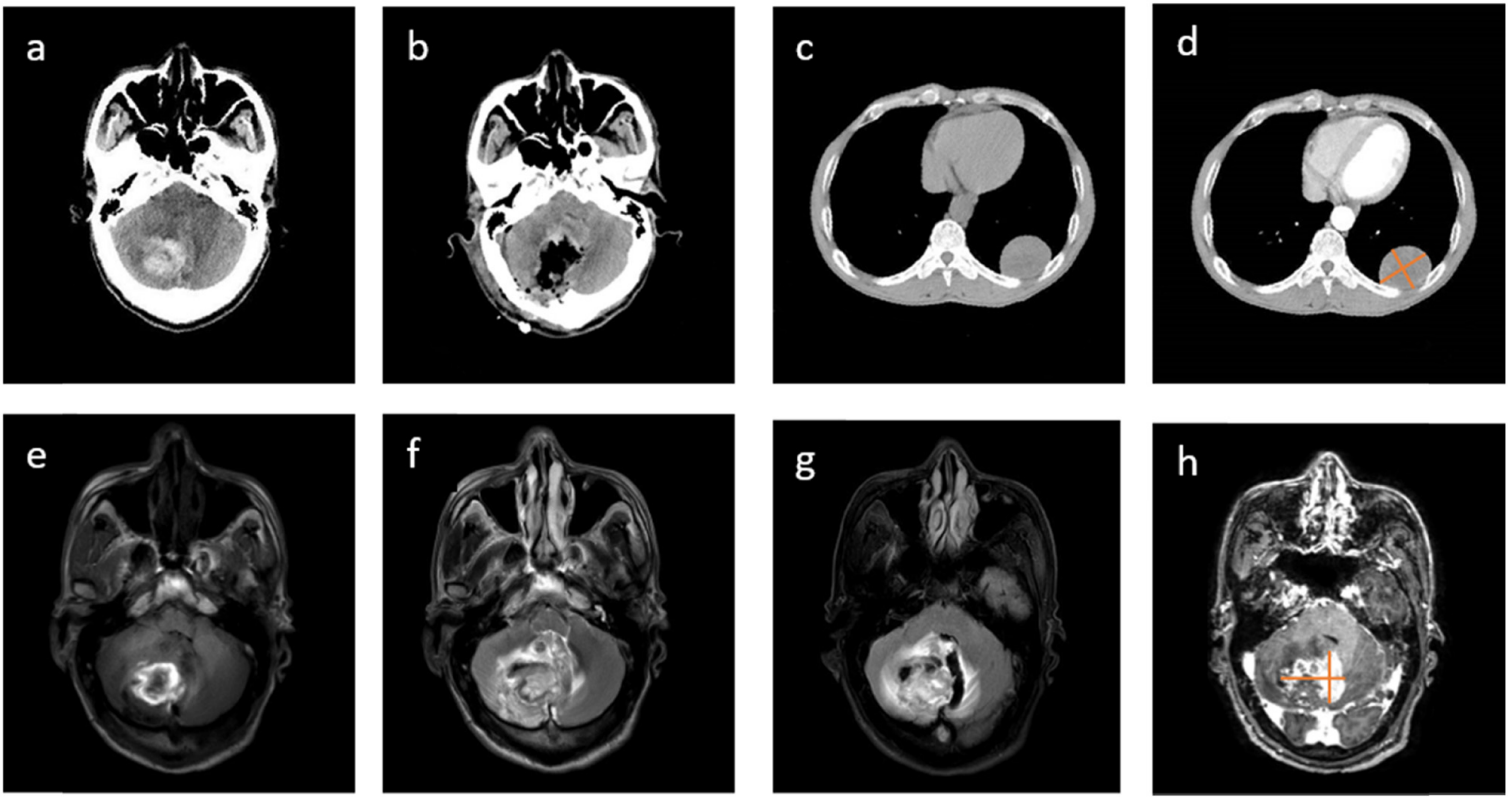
CT scan of the emergency room showed hemorrhage in the right cerebellar hemisphere (a). Postoperative CT scans revealed clear axial plane views of the surgically cleansed meningiomas and hematomas (b). (c) An image of a subpleural mass in the left lower lobe with well-defined, well-intact margins, and a slightly less uniform density. After enhancement scanning, there is a little heterogeneous enhancement of the chest occupation, tumor size is about 3.4 cm × 3.2 cm (d). The right cerebellar hemisphere exhibits a patchy confluence of T1-weighted, T2-weighted, and FLAIR signals (e–g); the peripheral T2-weighted edema signal has fuzzy boundaries. (h) After enhancement scanning, tumor tissue shows a faintly heterogeneous enhancement. The size of the tumor in the image is about 4.3 cm × 2.1 cm.
